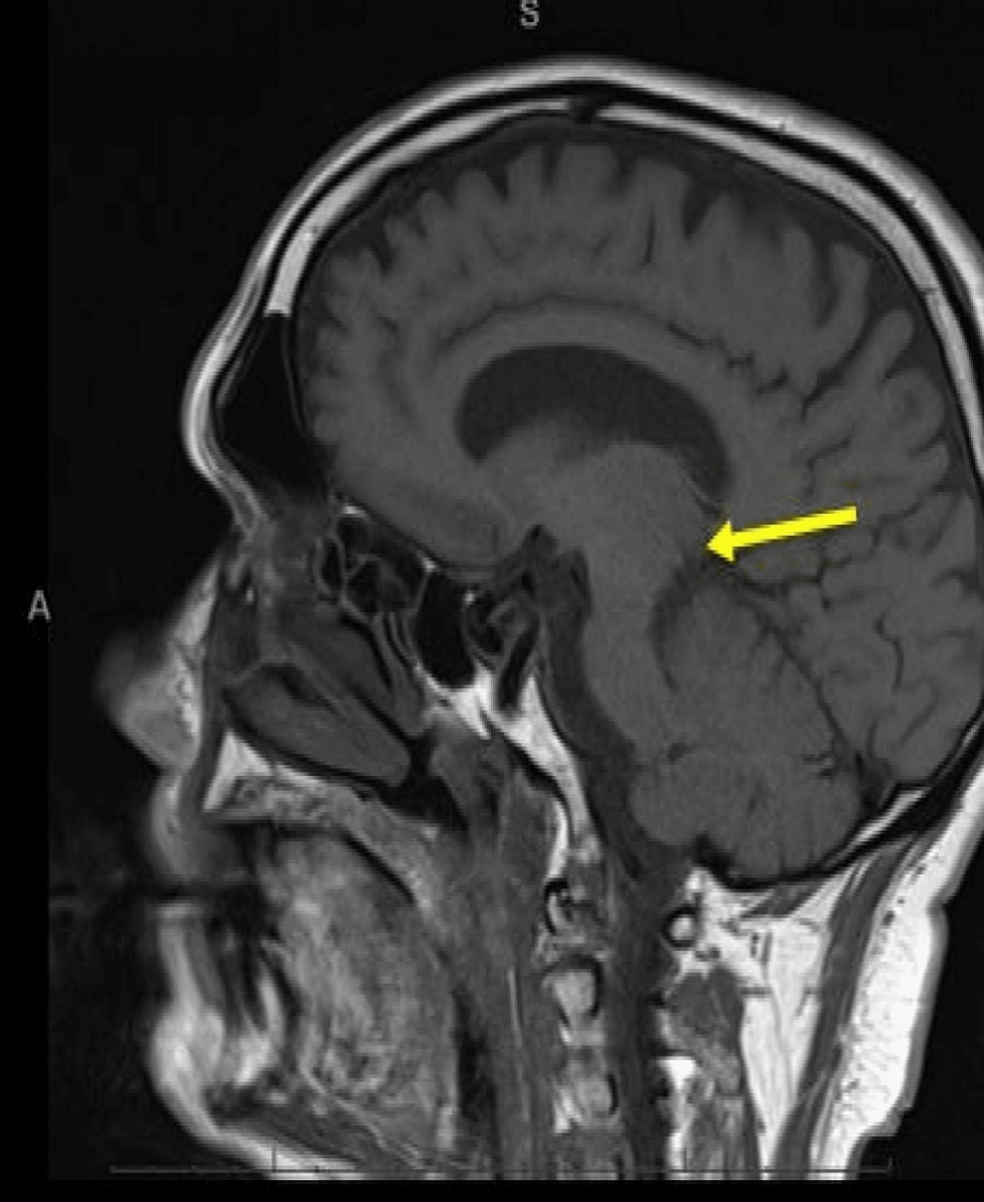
Magnetic resonance imaging of the brain (sagittal view): re-demonstration of restricted diffusion in the left paramedian tegmentum on diffusion-weighted imaging (yellow arrow).
